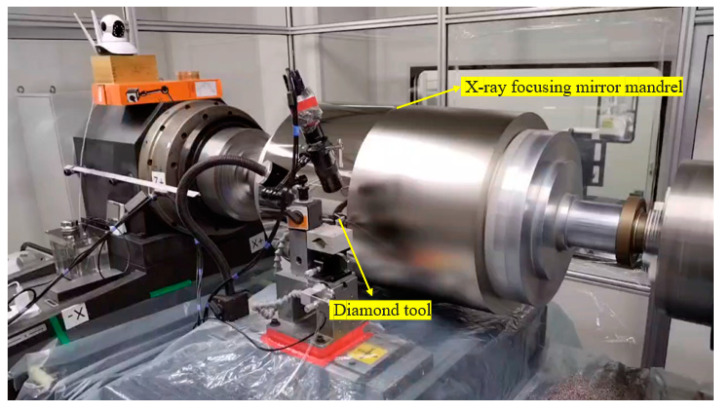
X-ray focusing mirror mandrel ultraprecision machining process.
